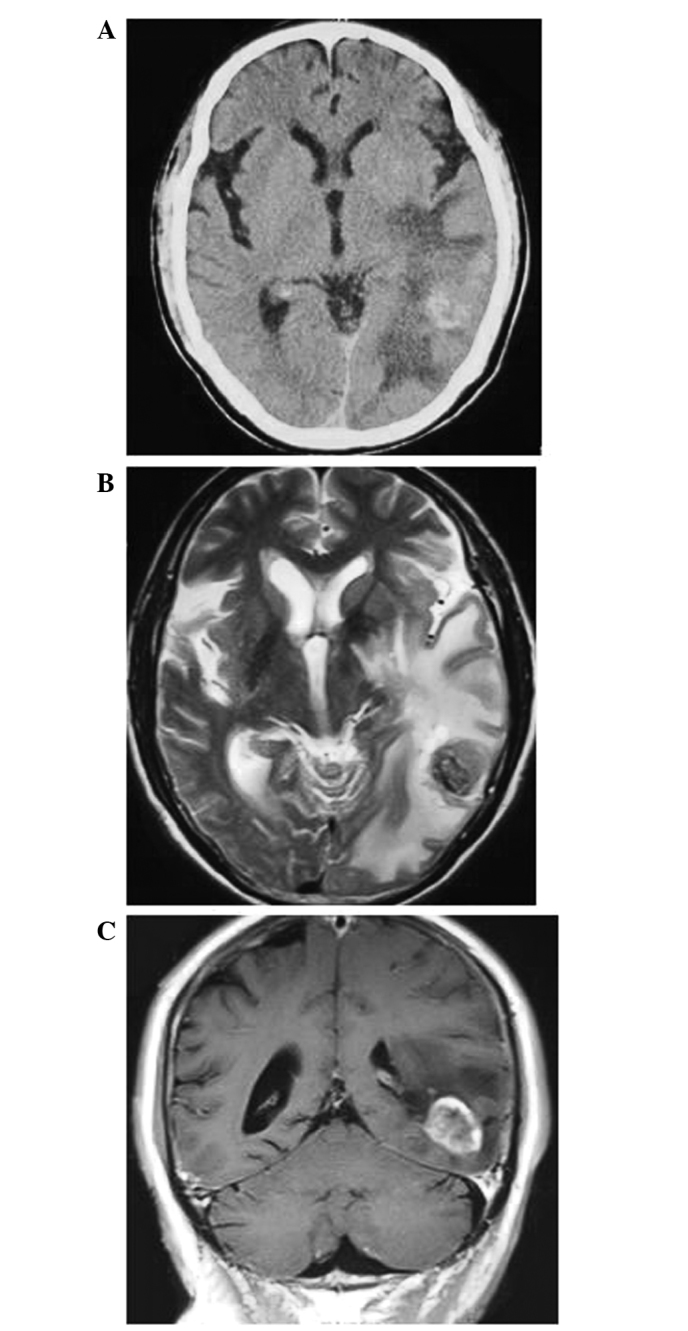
Prior to treatment, (A) axial CT, (B) axial MRI T2-weighted and (C) coronal MRI T1-weighted scans reveal brain metastasis in the left temporal lobe and cerebral edema located around the lesion. CT, computed tomography; MRI, magnetic resonance imaging.
